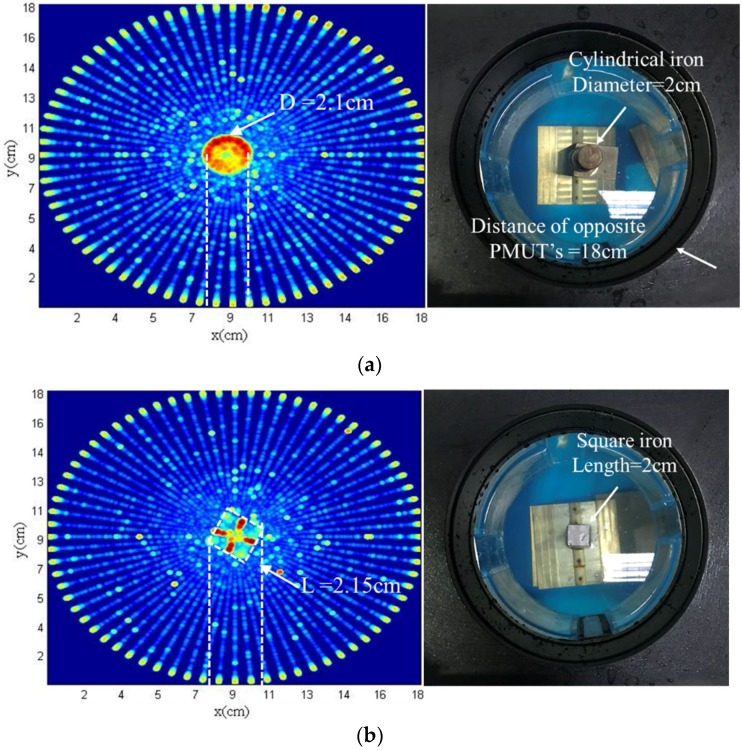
Ultrasonic tomography of iron using the ring system. (a) Cylindrical iron imaging and configuration; (b) Square iron imaging and configuration.
